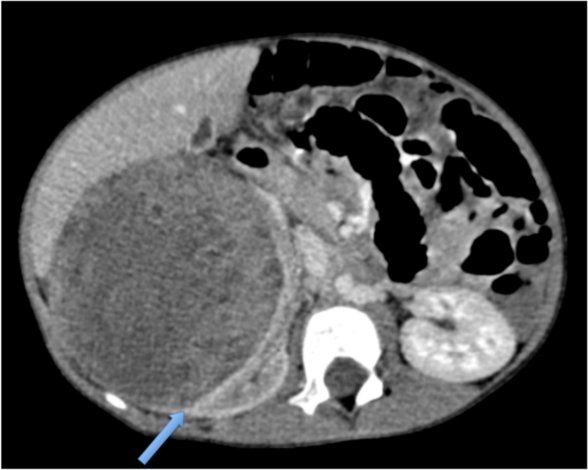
Axial CT of a 3 year old boy with right-sided Wilms tumour, again demonstrating the ‘claw sign’ (blue arrow).
